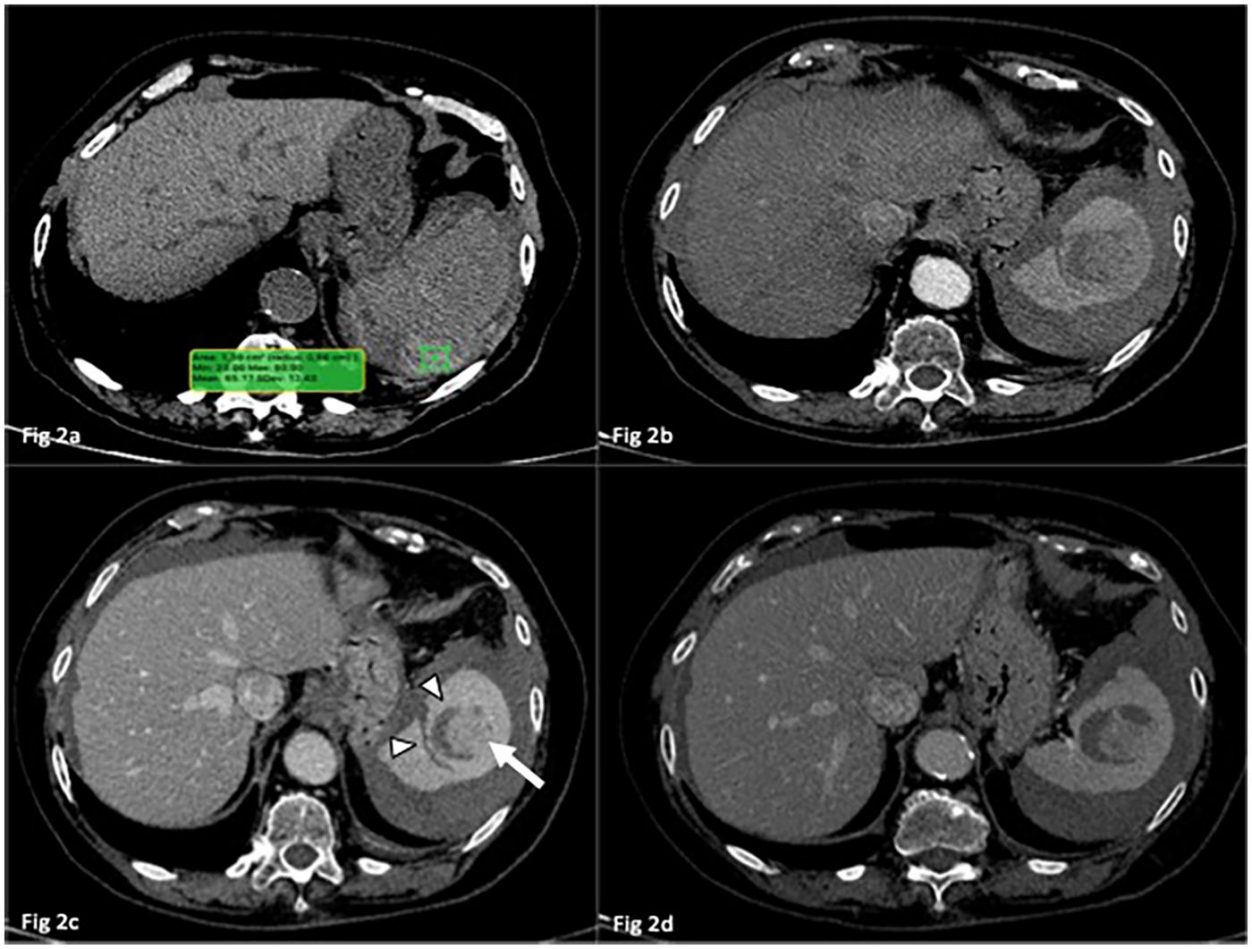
Axial CT scans. In (a) baseline CT scan confirms the presence of hemoperitoneum with sentinel clots in the perisplenic region (Region of interest ROI indicates 65HU, Hounsfield Unit). In (b–d) a well-defined hypodense laceration (arrowheads in c) is observed in continuity with a solid focal alteration (*arrow*), surrounded by a hypodense halo. These findings suggest that the rupture originated from the focal lesion, which may represent the structural substrate for SSR. In all enhanced phases, no evidence of active bleeding is noted.
